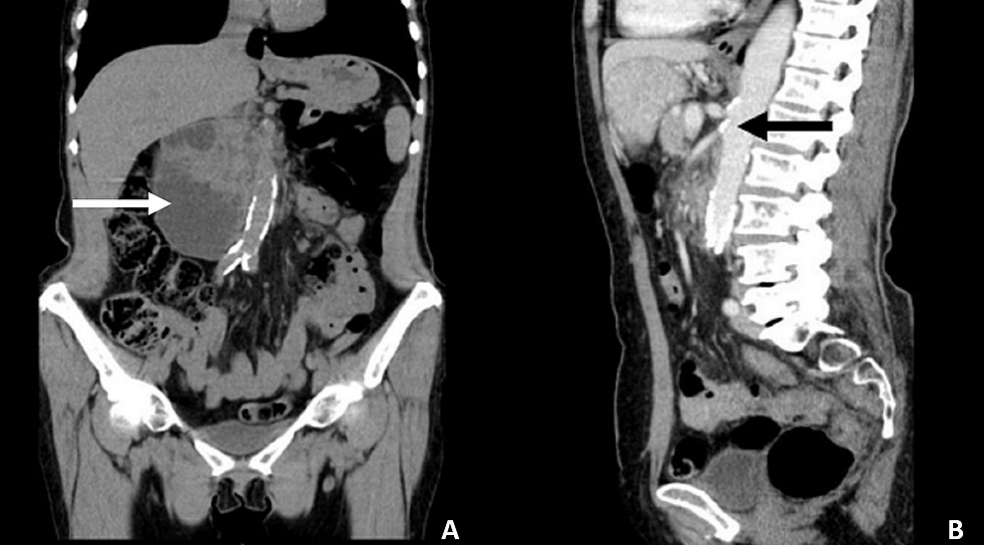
Contrast-enhanced abdominal-pelvic CT scan (A) Coronal view: white arrow evidencing duodenal arch dilation. (B) Sagittal view: black arrow signaling the aortomesenteric angle.
